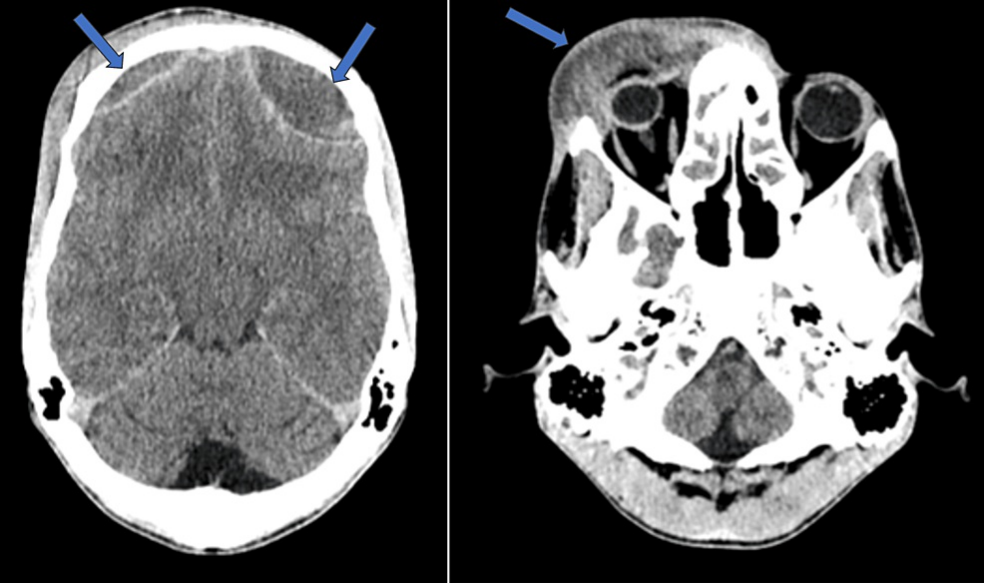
Orbital cellulitis and empyema in CT In the CT scan, collections are visualized in the preseptal, frontal, and right nasal dorsum regions, measuring approximately 7 × 1.7 cm in maximum axial and 5.7 cm in the longitudinal axis, extending into the intraorbital extraconal area adjacent to the orbit roof. The eyeball, extraocular orbital muscles, and optic nerve showed no abnormalities. Additionally, there was a bilateral epicranial intracranial collection with a maximum thickness of 2.4 cm, causing a mass effect on adjacent parenchyma and sulcus obliteration, associated with superior longitudinal sinus thrombosis. The anterior wall of the right frontal sinus showed signs of osteomyelitis. Furthermore, signs of chronic sinusitis were detected in the frontal sinuses, ethmoidal cells, and maxillary sinuses.
